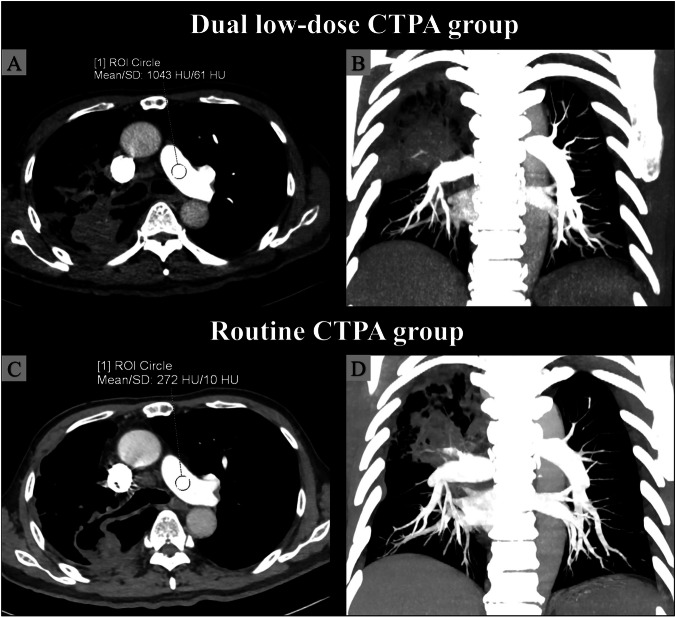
The axial contrast-enhanced CT images and MIP images in a 62-year-old male patient (BMI, 21.6 kg/m²) who underwent CTPA examinations 8 months apart using the VMI at 40-keV derived from dual low-dose CTPA (A) and routine CTPA at 100-kV (C) protocols. MIP images are generated from the corresponding axial CT data (B, D). The CT numbers measured in the pulmonary trunk are 1043.0 HU and 272.0 HU, respectively
